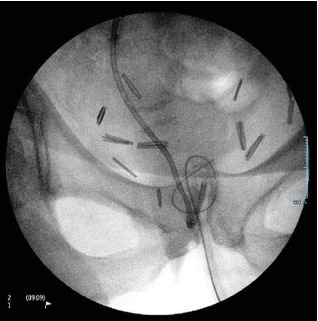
Intraoperative X-ray demonstrating maximal sensor wire insertion.
